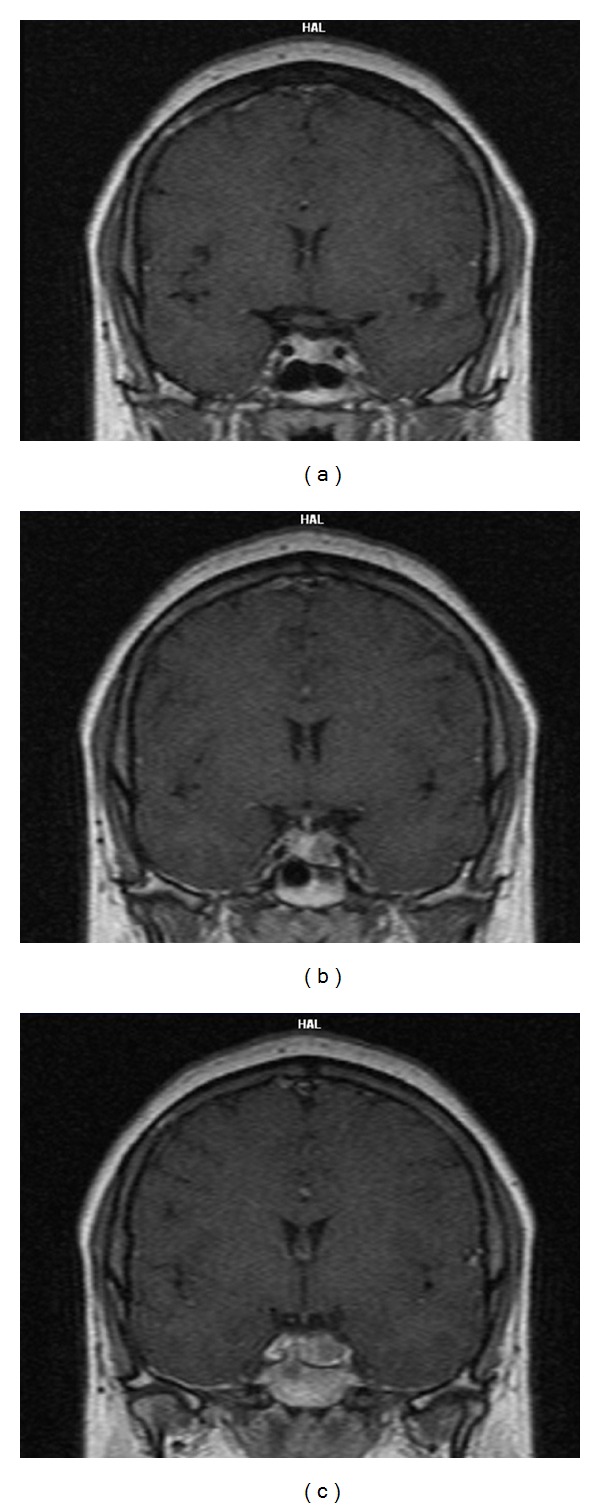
Postcontrast T1 weighted MRI after 1 year of cabergoline treatment: coronal sections at the levels corresponding to Figures 1(a), 1(b), and 1(c); a marked tumor shrinkage was seen (tumor size: 9 × 8 mm).
